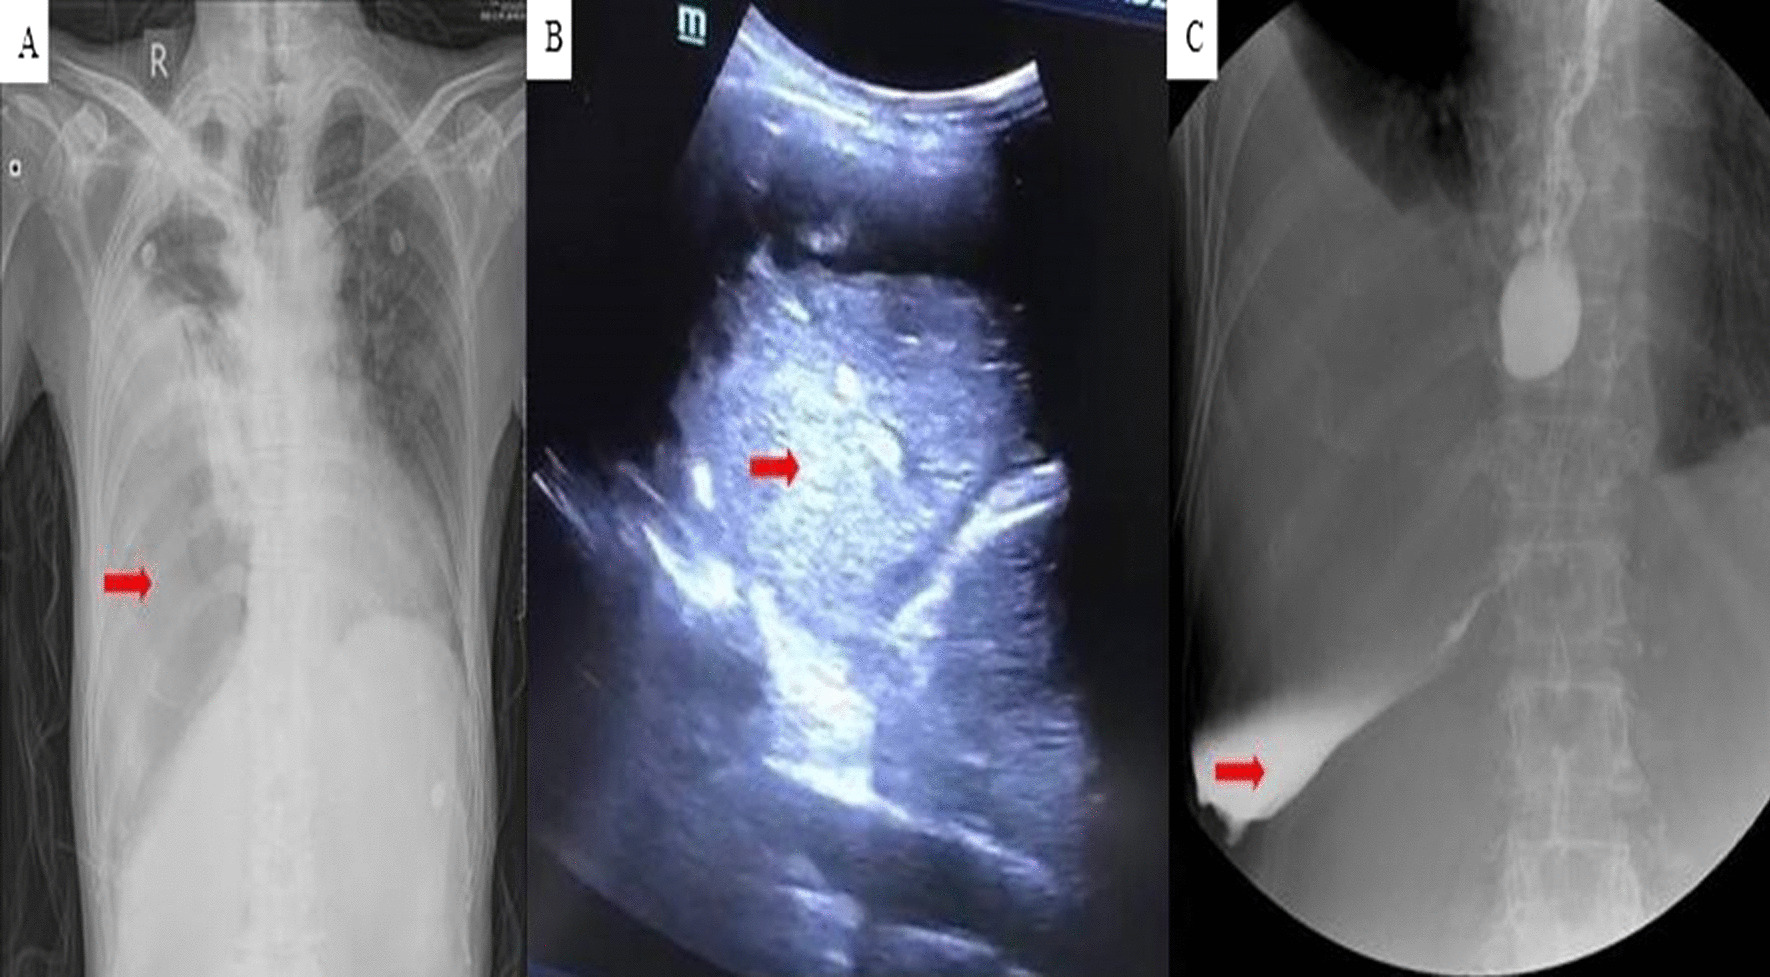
a Chest X-ray demonstrated a massive right hydrothorax (red arrow). b Chest ultrasound showed a floating hyper echoic mass (red arrow). c Upper gastrointestinal radiography gave us information that contrast agent was found in the chest cavity (red arrow)
